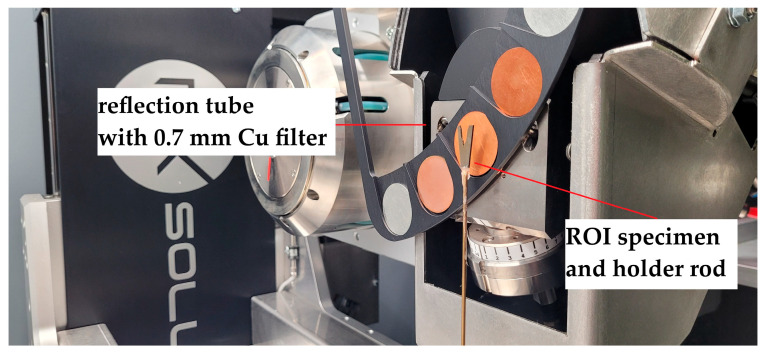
µ-CT measurement setup for ROI samples.
